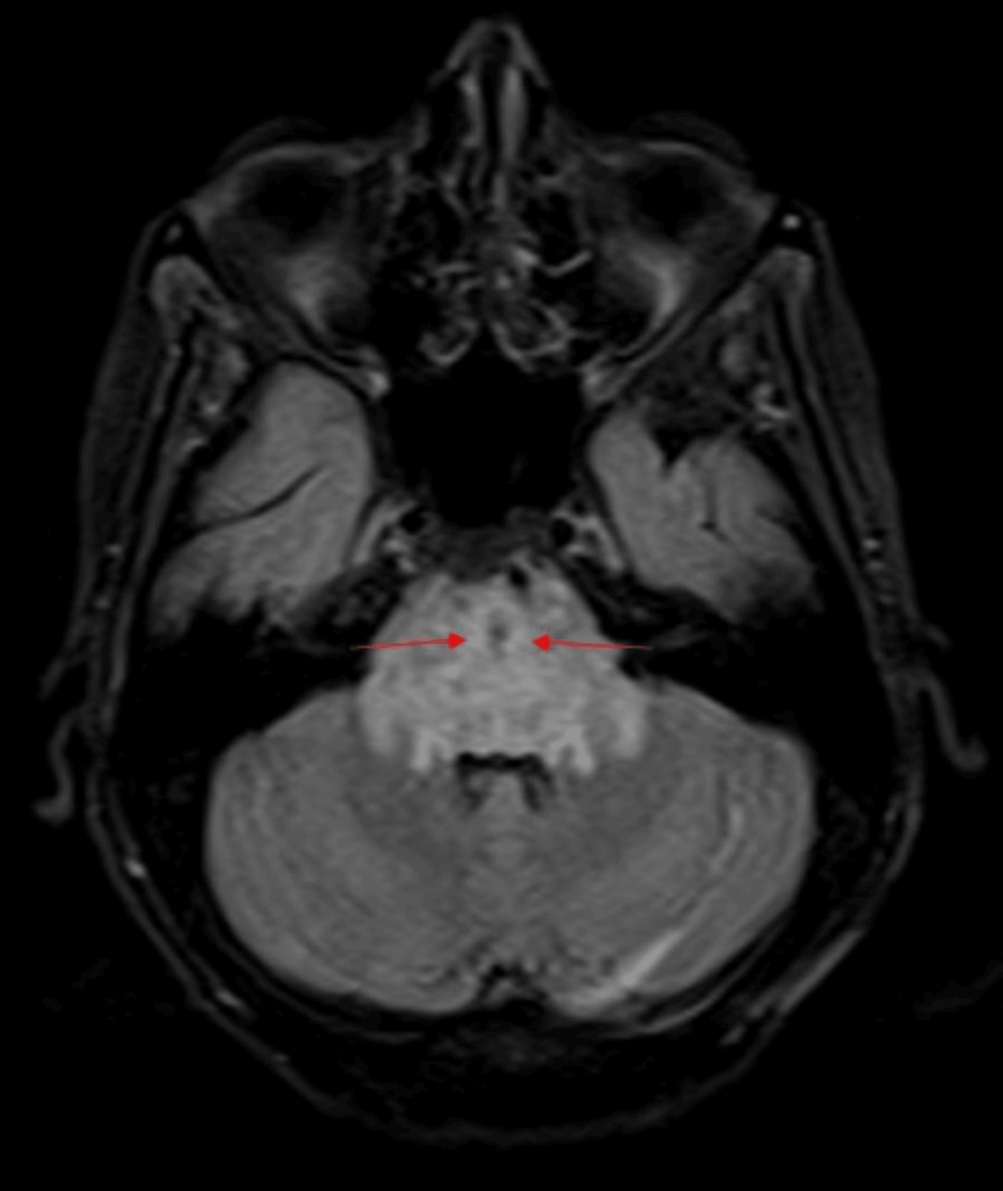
T2/FLAIR image showing swollen pons with hyperintense signals in central pons. There is perifocal edema involving the midbrain, posterior limb of the internal capsule superiorly, medulla inferiorly, and middle cerebellar peduncles posterolaterally. FLAIR: fluid-attenuated inversion recovery
